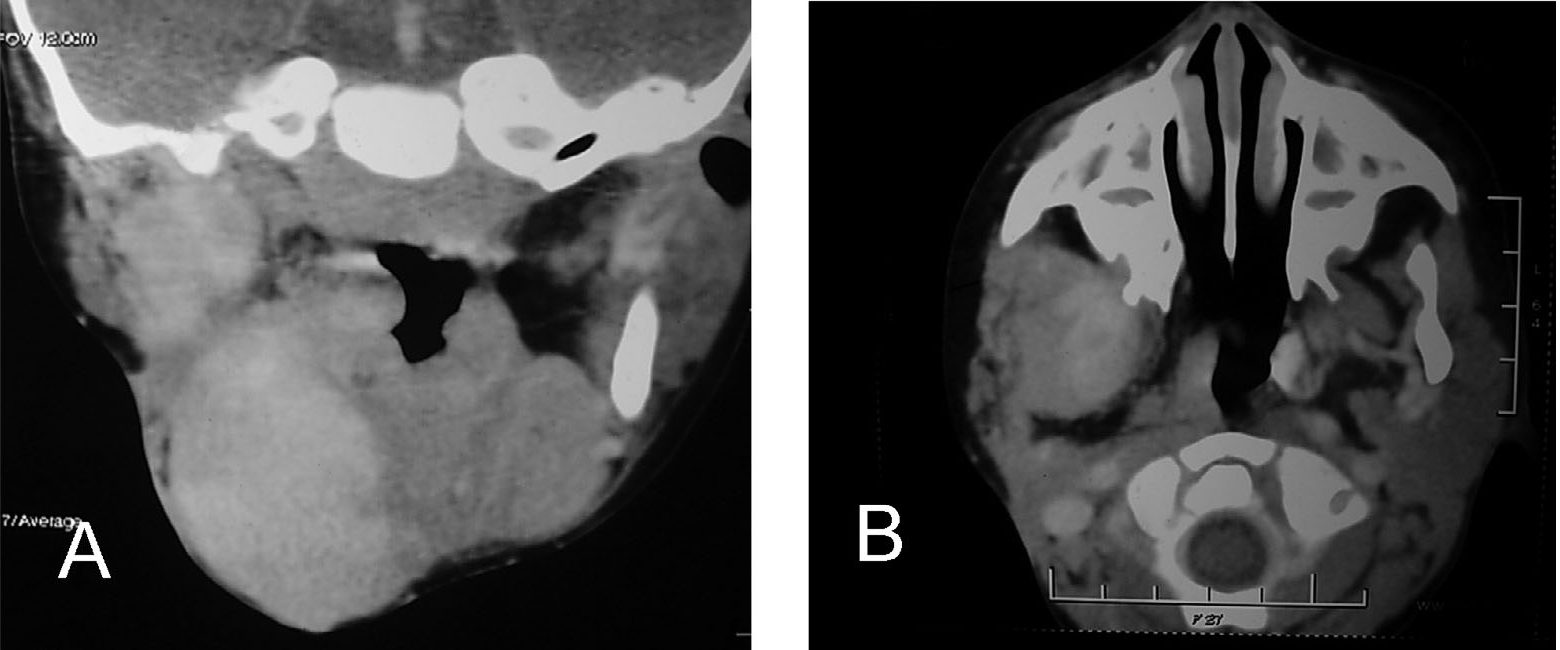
A CT scan revealing recurrence at the floor of mouth and presence of tumor in the infratemporal fossa and base of skull.
